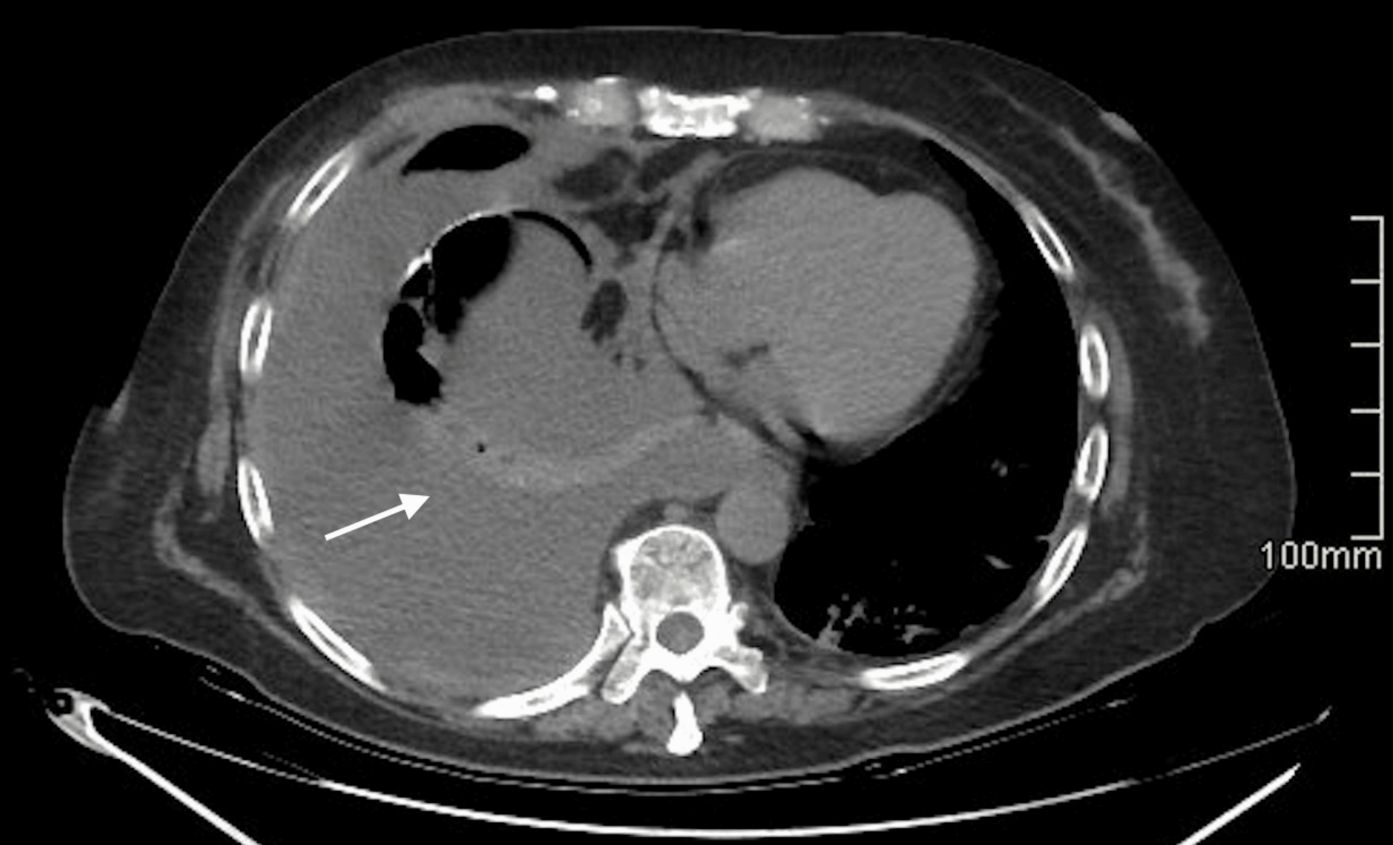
A non-contrast thoracic CT scan identified moderate right pleural effusion
